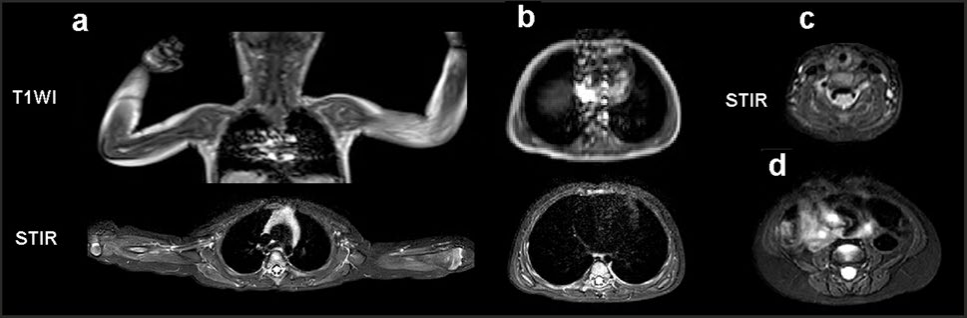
MRI of the shoulder girdle muscles of a 1.8-month-old proband (T1, STIR). a: shoulder girdle muscles (T1, STIR); b: trunk muscles at the level of Th6-7 (T1, STIR); c: neck muscles (STIR); and d: trunk muscles at the level of L5 (STIR)
